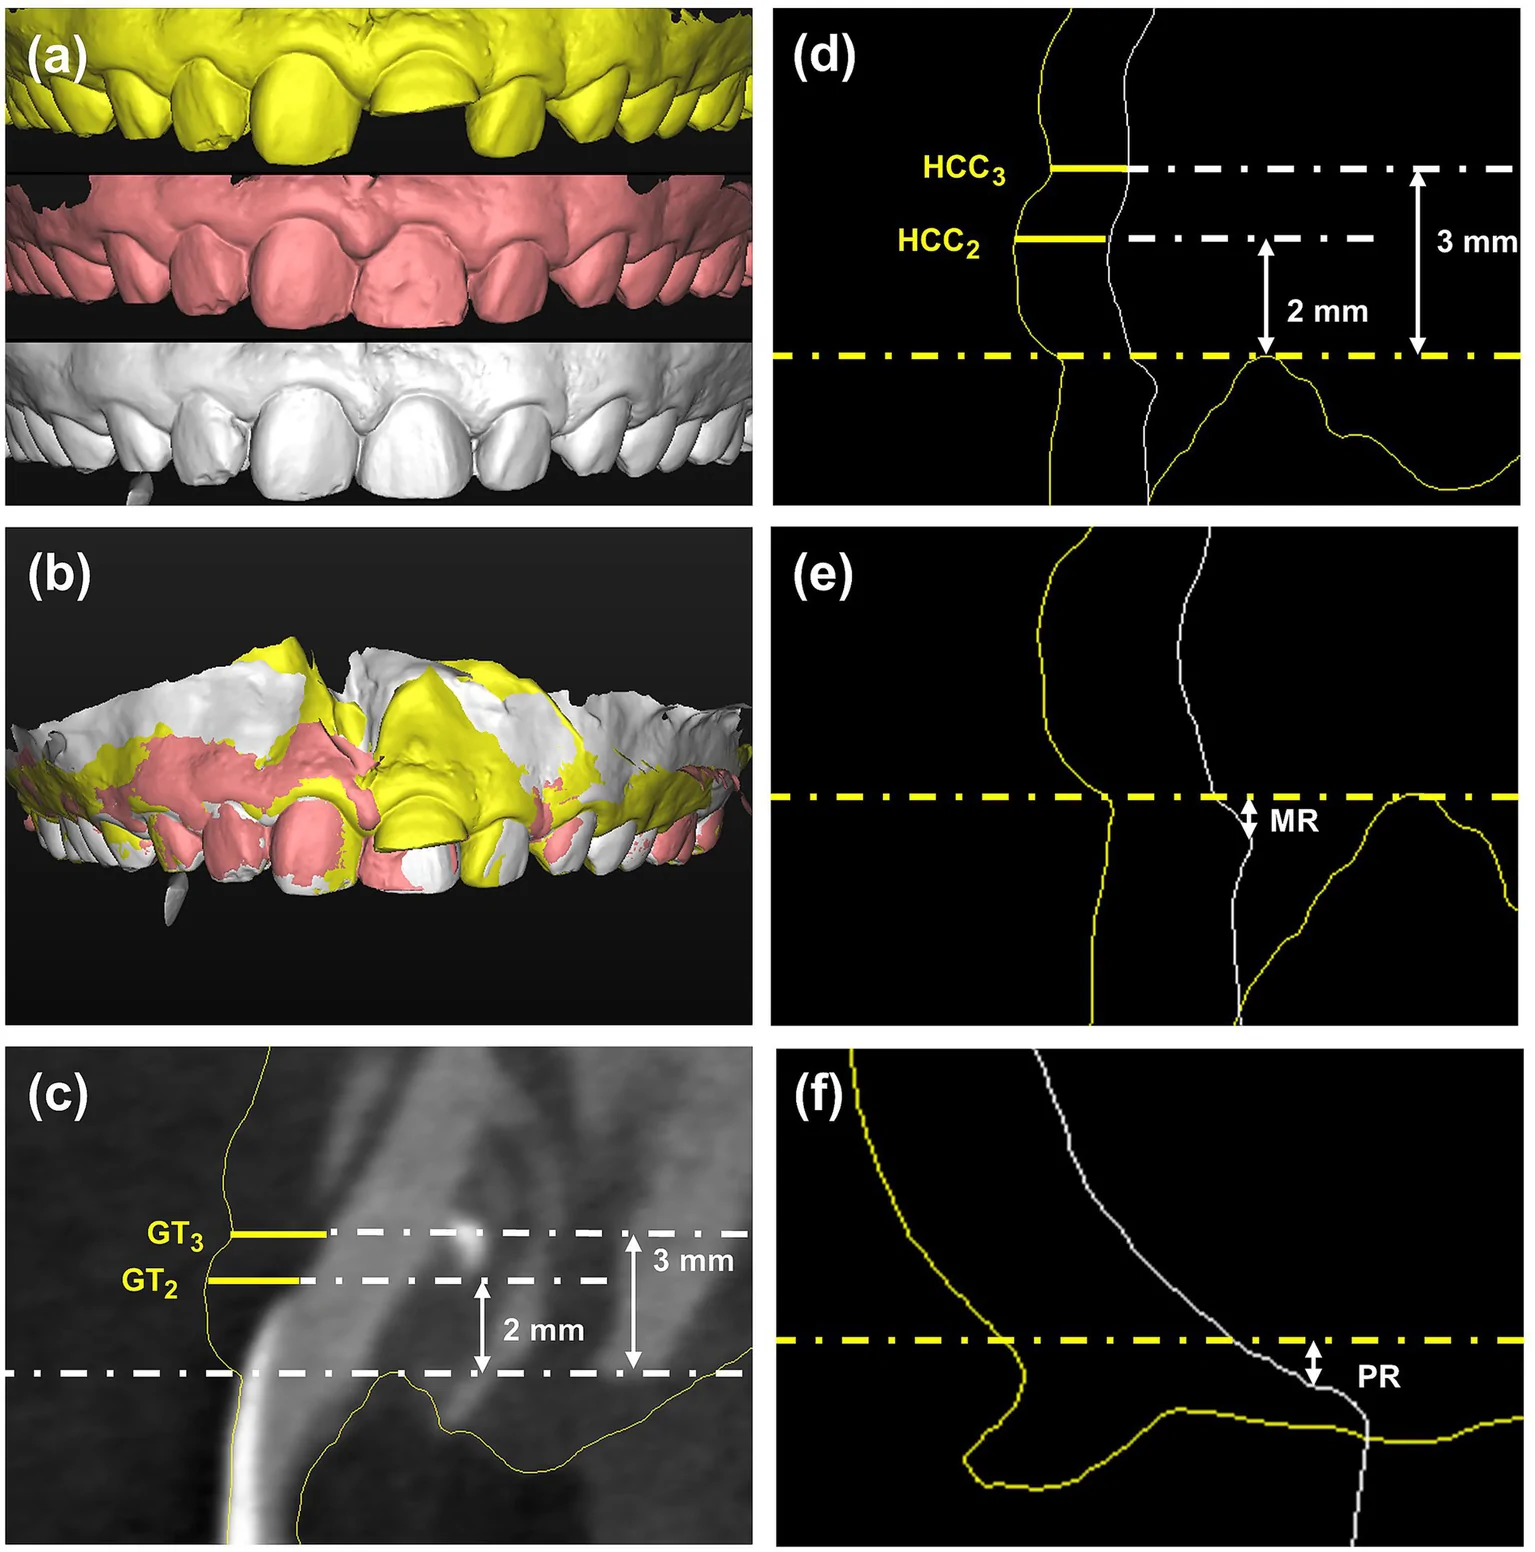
(a,b) STL files of the intraoral scan at T_1_ (red) and T_2_ (white) were superimposed on the intraoral scan at T_0_ (yellow). (c) Gingival thickness (GT) was measured as the horizontal distance between the contour of T_0_ (yellow) and the tooth surface of the preoperative CBCT images measured at two levels (2 and 3 mm apical to the gingival zenith at T_0_: GT2 and GT3). (d) Horizontal contour collapse (HCC) was measured as the horizontal distance between the contour of T_0_ and T_1_/T_2_ at two levels (2 and 3 mm apical to the gingival zenith at T0: HCC_2_ and HCC_3_). (e) The midfacial recession (MR) was measured as the vertical distance between the gingival zenith at T_0_ and T_1_/T_2_. (f) The papilla recession (PR) was measured as the vertical distance between the top of the papilla at T_0_ and T_1_/T_2_. According to the location of the papilla, the PR was labeled as mesial papilla recession (MPR) and distal papillary recession (DPR).
